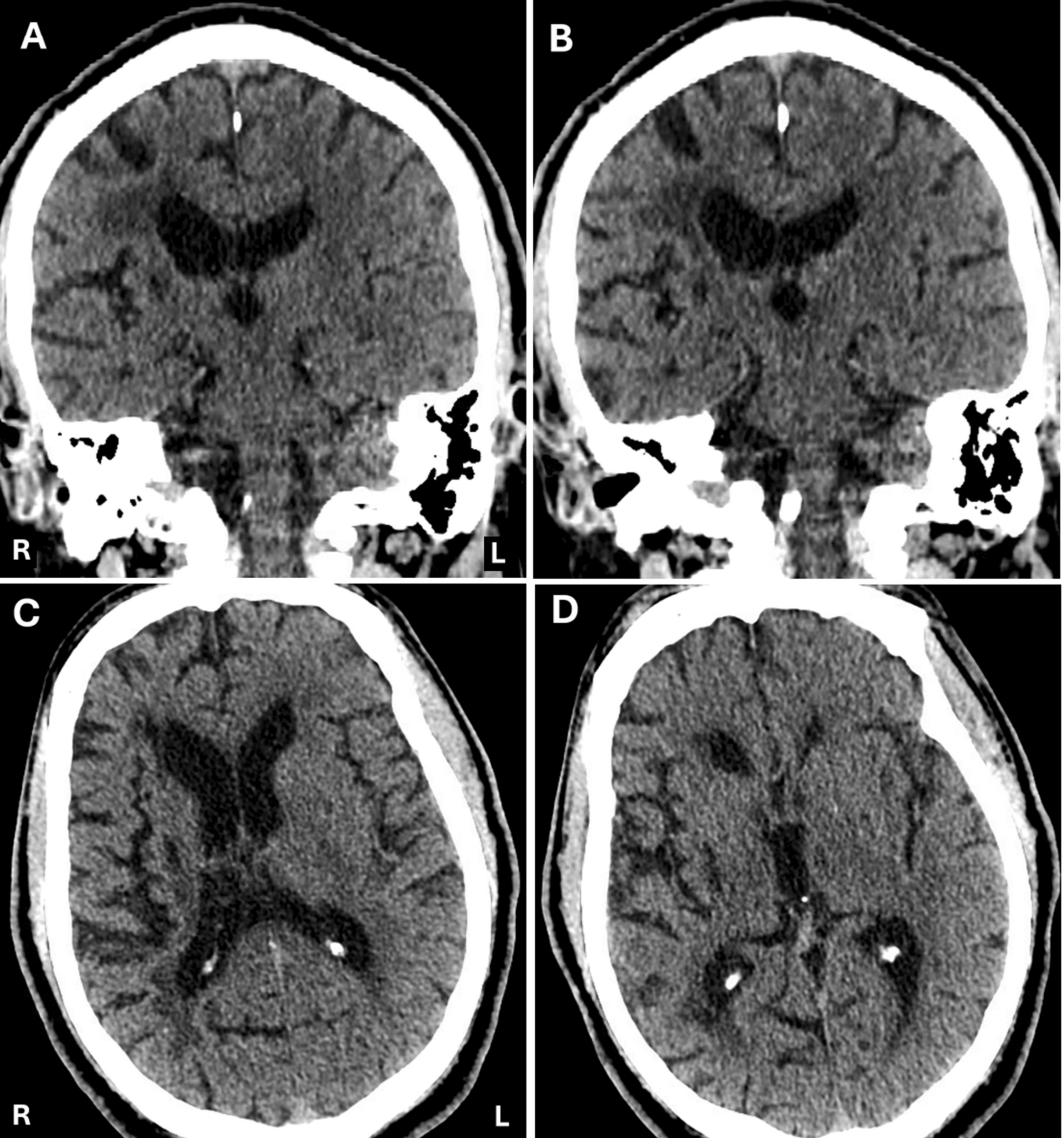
CT Head without contrast performed in the ED No acute intracranial abnormalities were seen in the coronal (A, B) or transverse (C, D) images.
